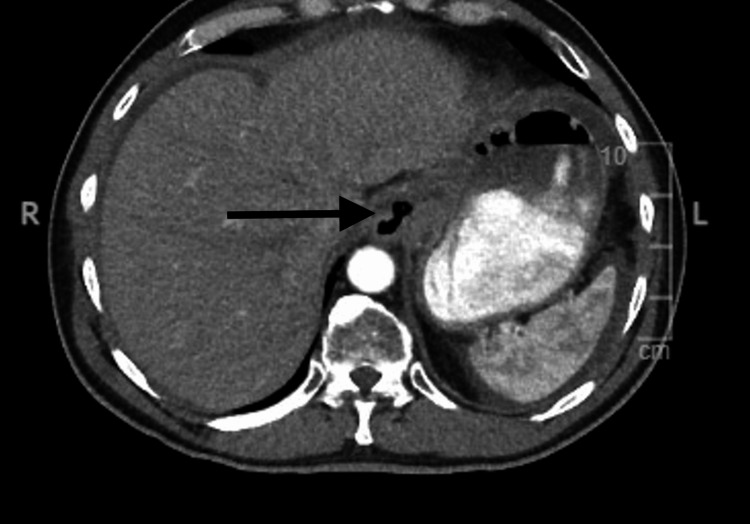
Abdominal CT scan showing thickening of the distal esophagus (black arrow) consistent with malignant disease
